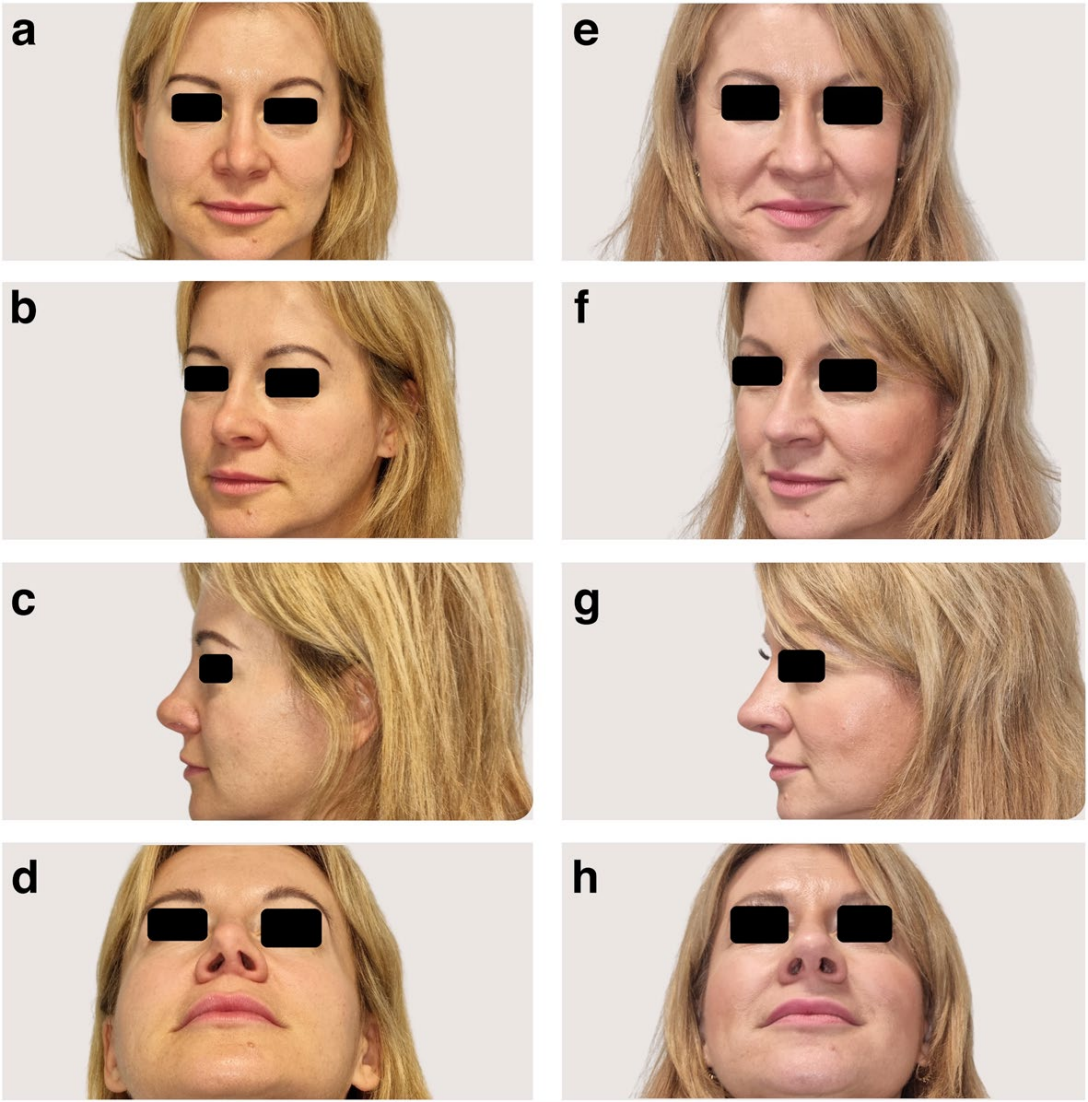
CT-assisted and 3D-assisted revision rhinoplasty with costal graft in patient B with severe functional and aesthetic issues after previous rhinoplasty. a–d Pre- and e–h postoperative photos
